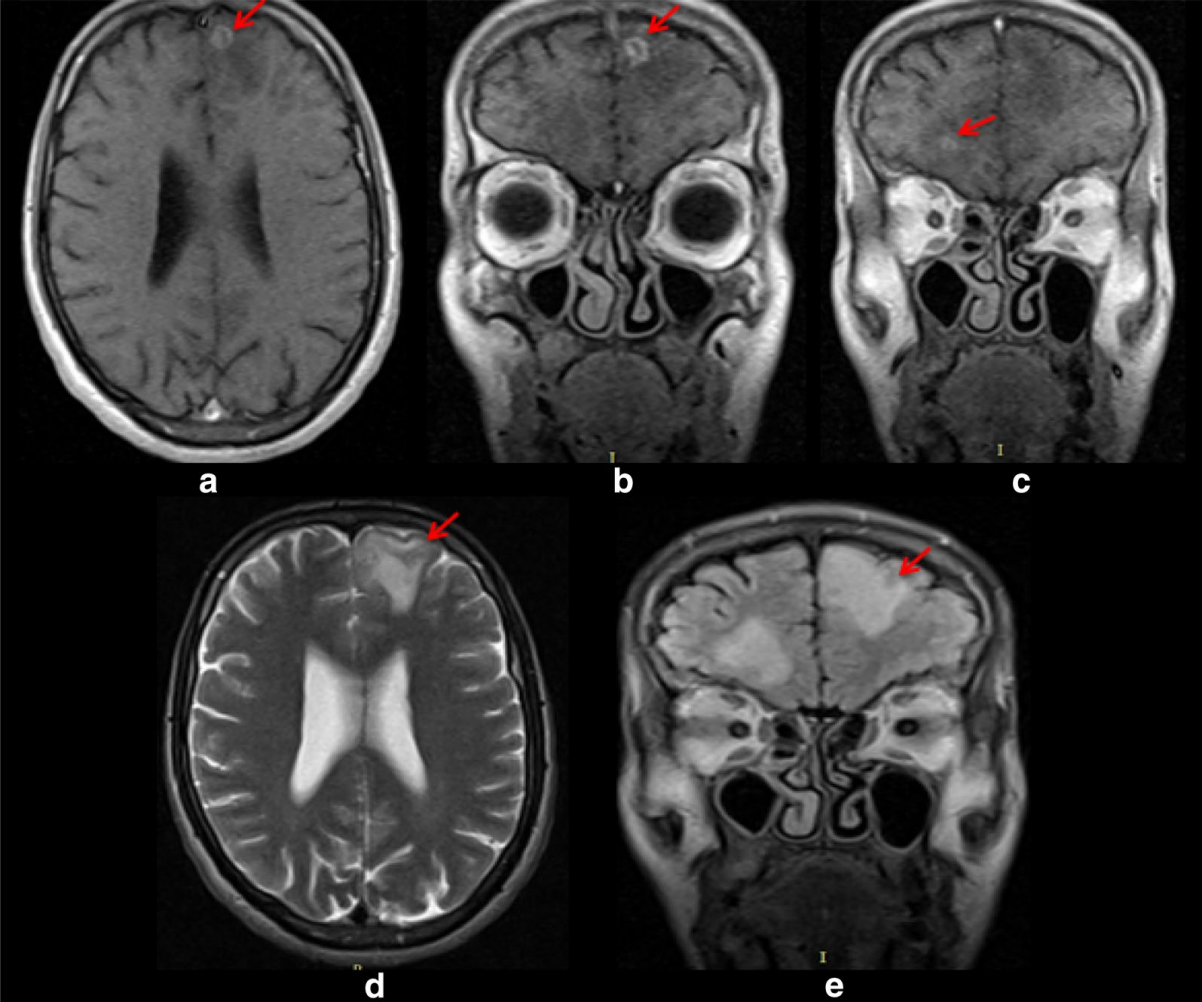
a–e: Post contrast T1 axial (a) and coronal brain MRI showing ring enhancing lesions in the left (b) and right (c) frontal lobal region. T2 axial (d) and coronal FLAIR (e) images showing perilesional edema (red arrows)
